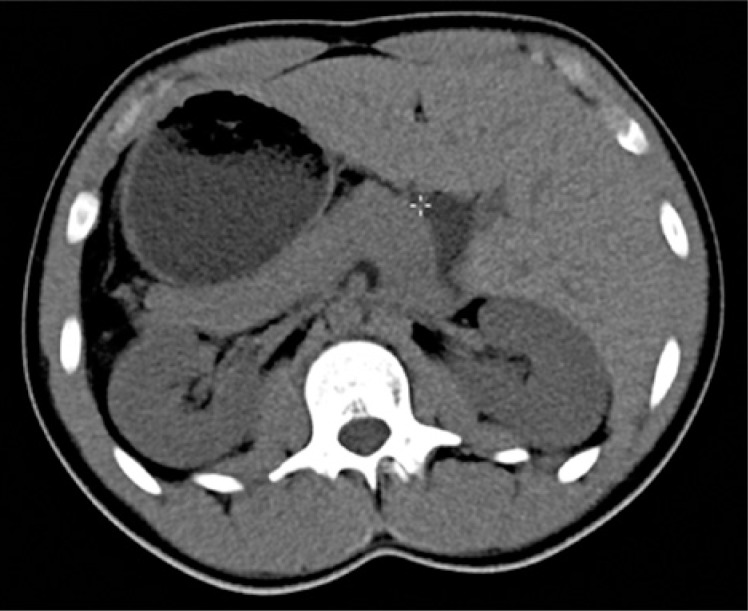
Computed tomography of the total abdomen in cross-sectional view showing abdominal organs in a mirrored position relative to their normal topography
